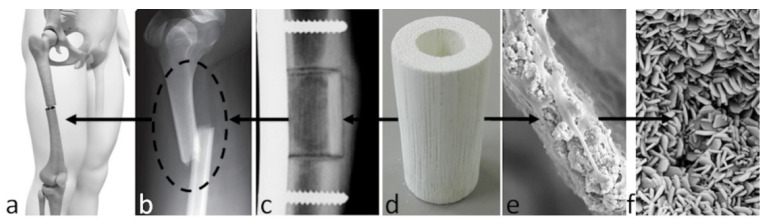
Biomorphic scaffolds for long bone regeneration and their application in long bone defects: (a) example of implantation site; (b) critical size defect; (c) radiography of implanted scaffold; (d) bone scaffold from wood; (e) scaffold–cell interaction, 250×; (f) scaffold nanostructure, 25,000×.
